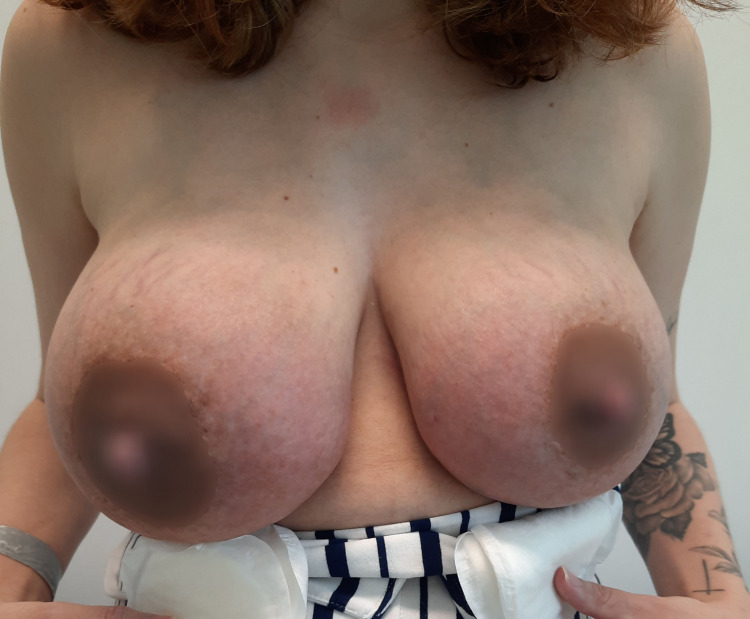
Day five of continuous therapeutic ultrasound
